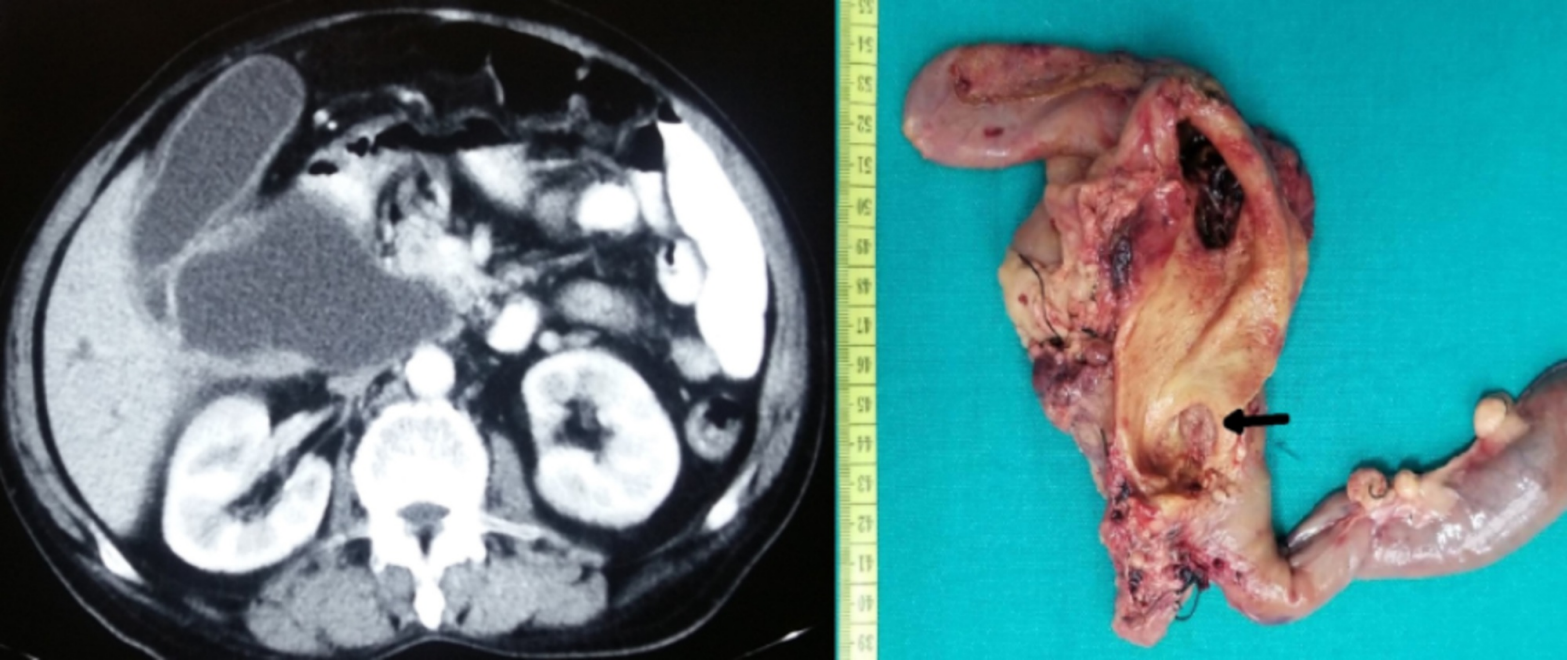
CT (cystic dilation of extrahepatic bile duct - 6 cm) and intraoperative image of pancreatoduodenectomy for choledochal cyst with malignancy (distal cholangiocarcinoma - arrow)
